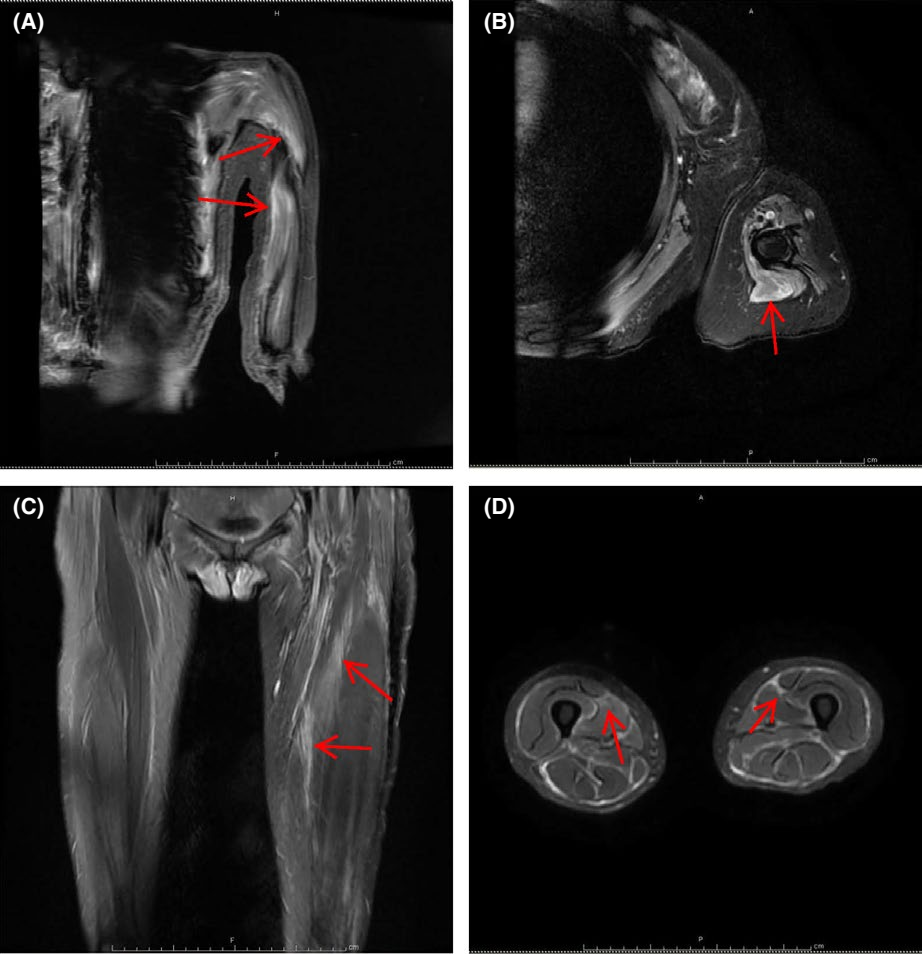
MRI Imaging. T2‐weighed Imaging Showed High Intensity in the Biceps, Deltoid, and Triceps Muscles (A & B from the CASE 1), and in Muscles and Fascia of the Thigh (C & D from the Case 2)
